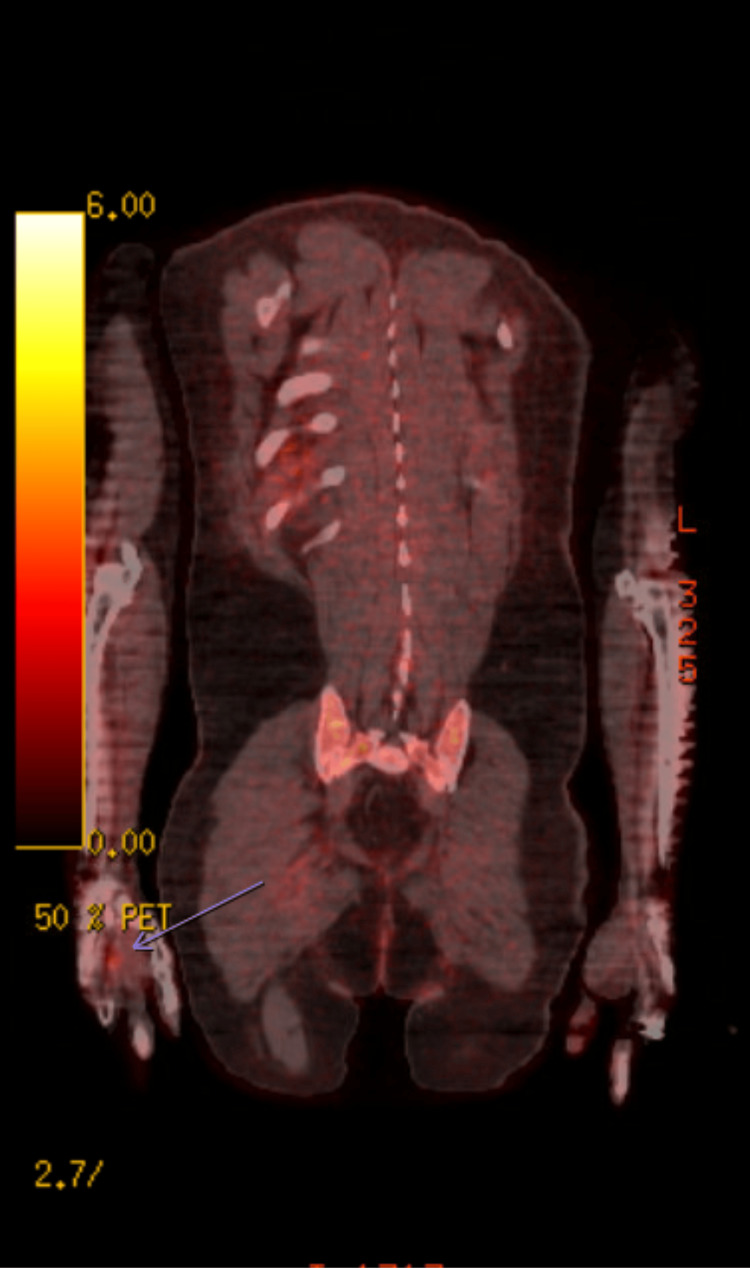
Faint focal FDG activity within the right third metacarpal visualized on the reinterpretation of PET/CT FDG: fluorodeoxyglucose; PET/CT: positron emission tomography/computed tomography.
